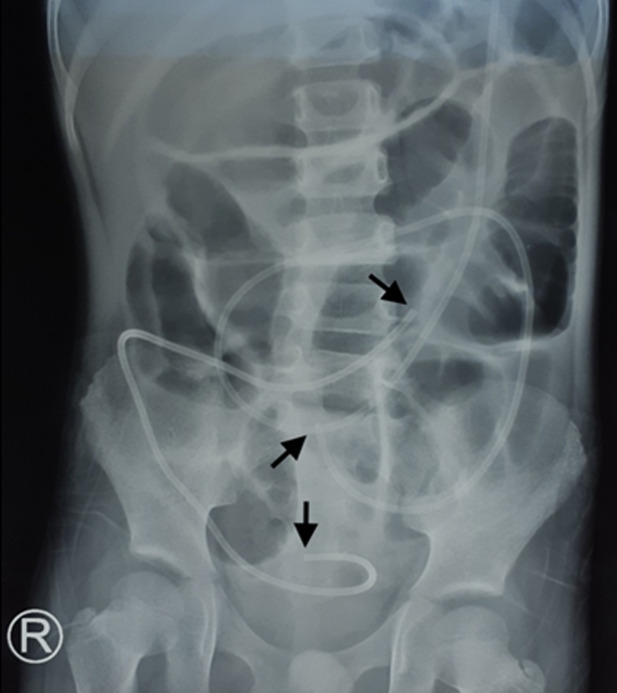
plain abdominal X-ray showed of fractured ventriculoperitoneal shunt tip in abdominal cavity
